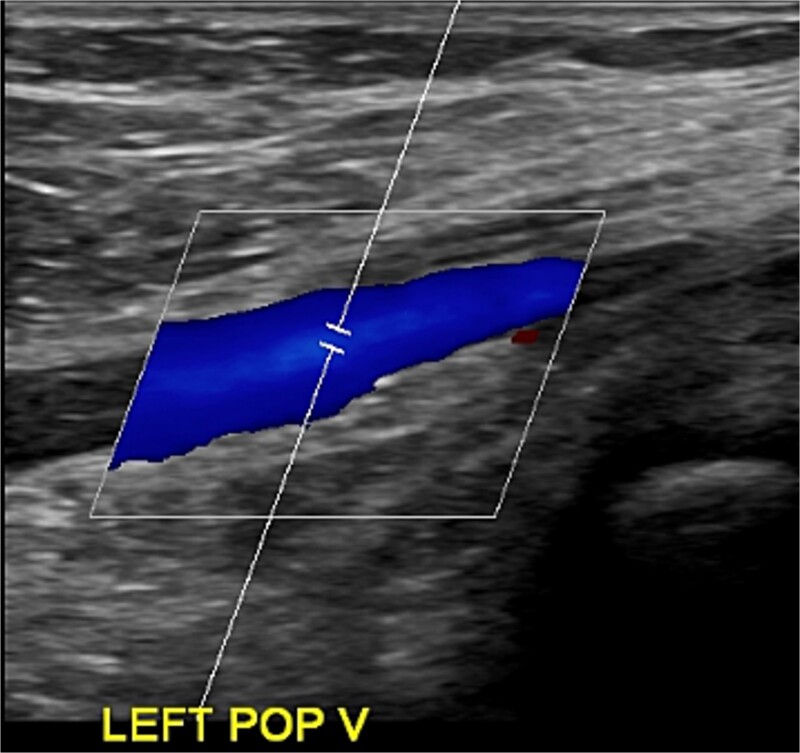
Ultrasonography obtained in January of 2023 demonstrating resolution of DVT.
